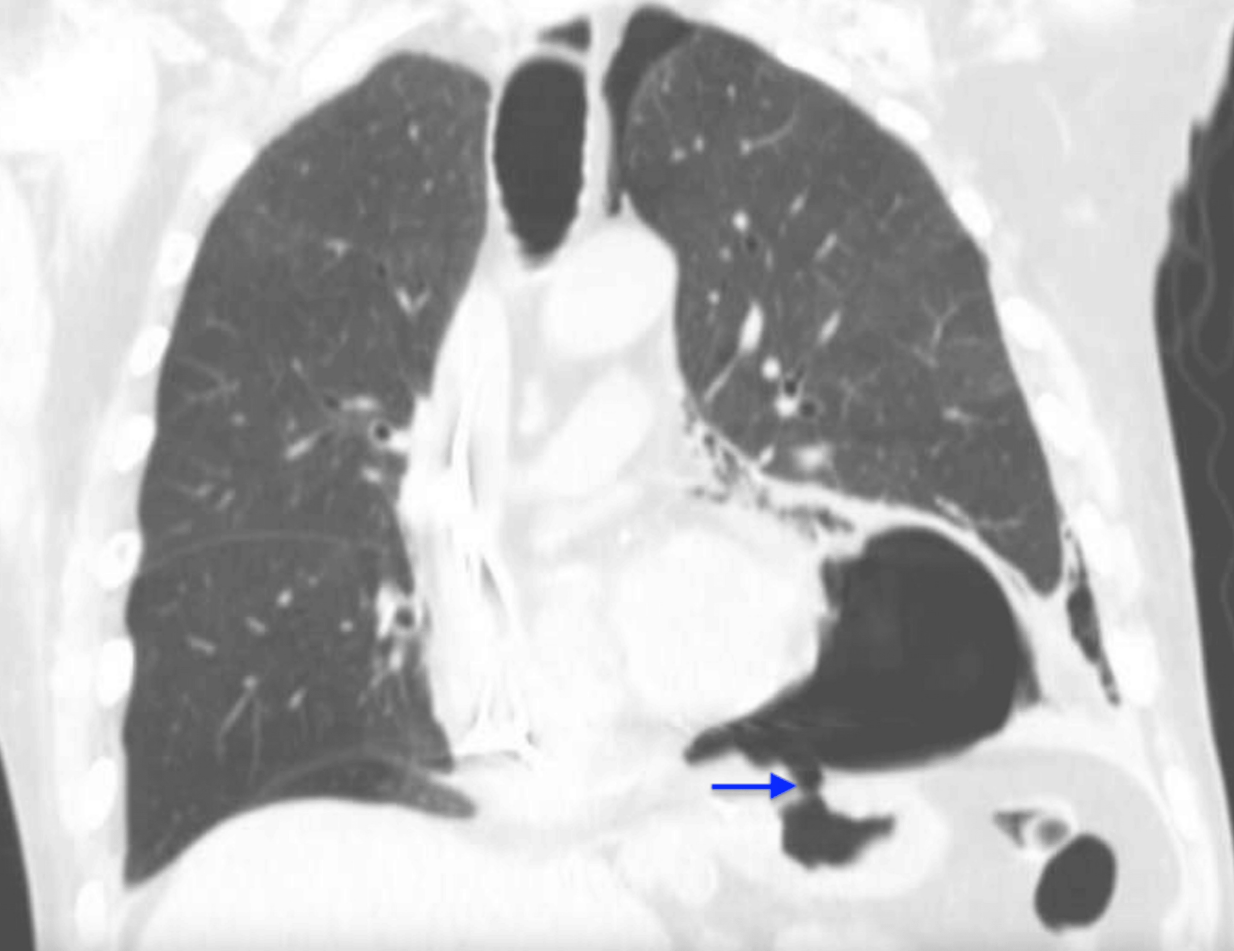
Coronal chest CT scan from 2019 CT scan demonstrates pneumopericardium and a second GPF (arrow). GPF, gastropericardial fistula
